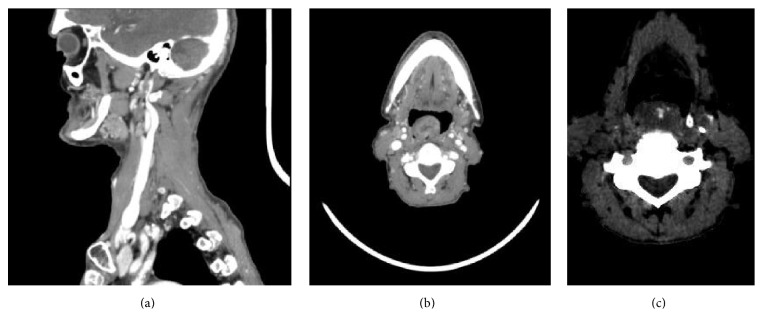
CT scans of the patient. (a) Sagittal view of head and neck. (b, c) Axial views.
